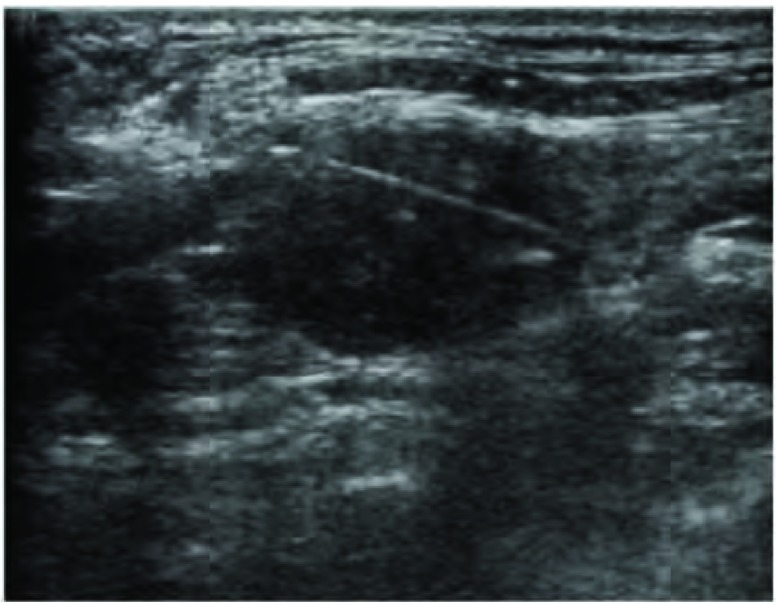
超声引导下穿刺活检，肿大的锁骨上淋巴结内可见清晰的穿刺针道。 Puncture biopsy guided by ultrasound. We can see needle path in the supraclavicular lymph node clearly.
